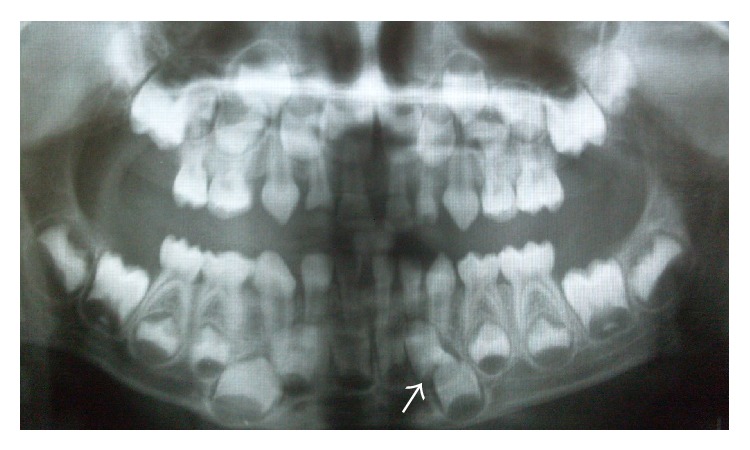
Panoramic radiograph of the patient's brother showing impacted supernumerary tooth.
